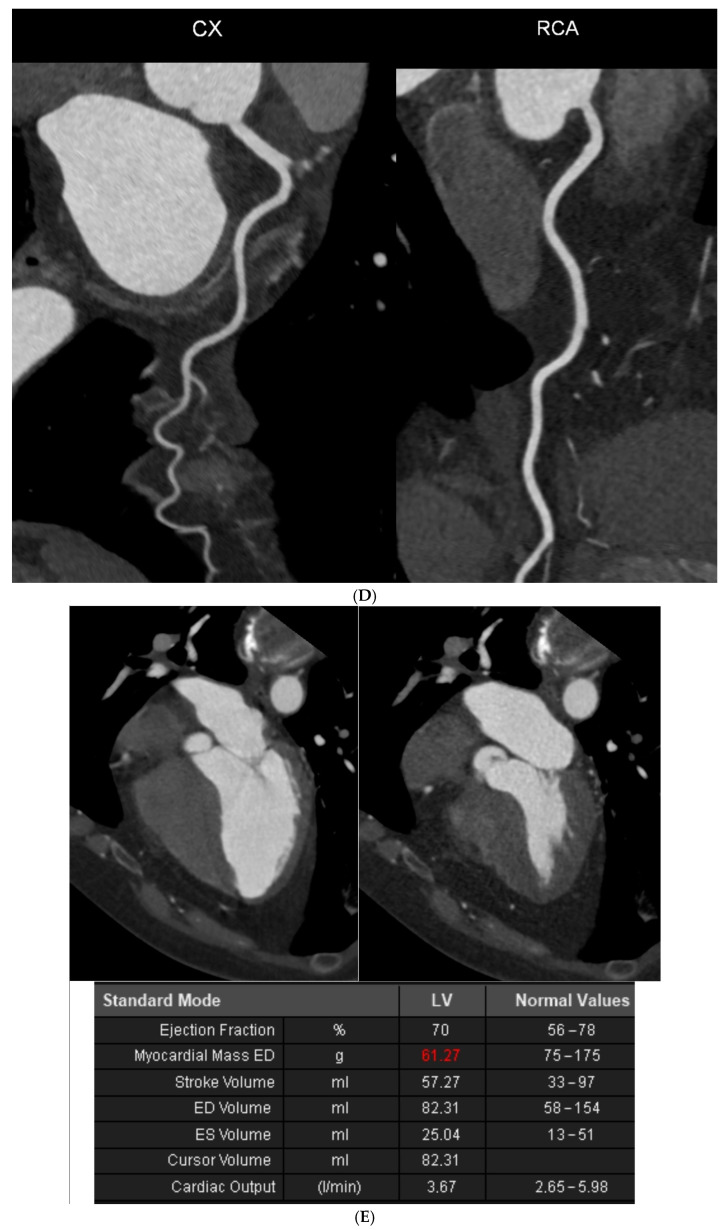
Case 1. Coronary artery calcium score (CACS) = 0 and significant coronary artery stenosis in cardiac computed tomography angiography (CCTA). A 70-year-old female patient was referred for coronary computed tomography angiography via the outpatient cardiology clinic due to reported non-specific chest discomfort and numerous risk factors for cardiovascular diseases in their medical history. CCTA examination was performed according to the standard protocol. (A) In the native phase of the CCTA examination, no calcified changes were detected in the topography of the coronary arteries. (B) Computed tomography volumetric reconstruction technique. Good-quality images were obtained from the angiographic phase of the CCTA examination. (C) Curved multiplanar reconstructions (cMPR). In the middle section of the left descending artery (LAD), at the level of the second diagonal branch (Dg2), a large non-calcified atherosclerotic plaque is visible. Mid-LAD stenosis was estimated to be approximately 70%. The entire CCTA met 4 CAD-RADS (coronary artery disease reporting and data system) criteria.). (D) Curved multiplanar reconstructions (cMPR). The left main (LM), left circumflex (LCx), and right coronary artery (RCA) are visualized; these were patent and without stenoses.). (E) Assessment of left ventricular functional parameters. The left ventricular ejection fraction was 70%, the left ventricular end-diastolic volume was 82.31 mL, and the left ventricular mass was 61.27 g. The left ventricle was not enlarged and had normal global systolic function. In further diagnostic and therapeutic procedures, invasive coronary angiography confirmed the presence of significant LAD stenosis. Percutaneous coronary intervention with stent implantation in the LAD was performed.
